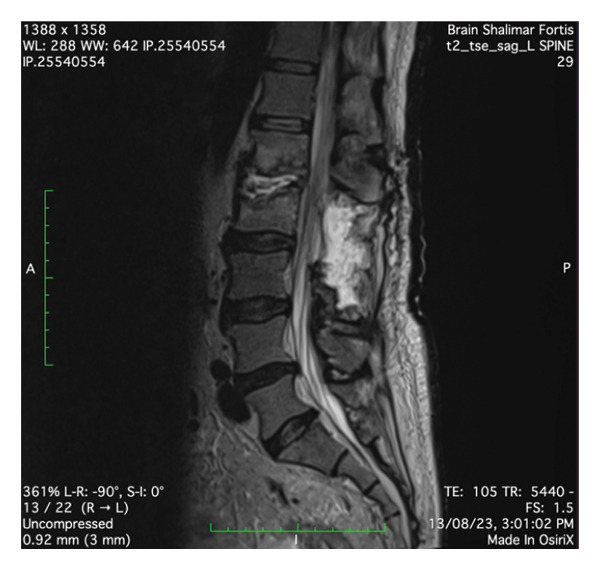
Postoperative T2‐weighted MRI images following L3 laminectomy and drainage of the L3‐L4 anterior epidural abscess.
